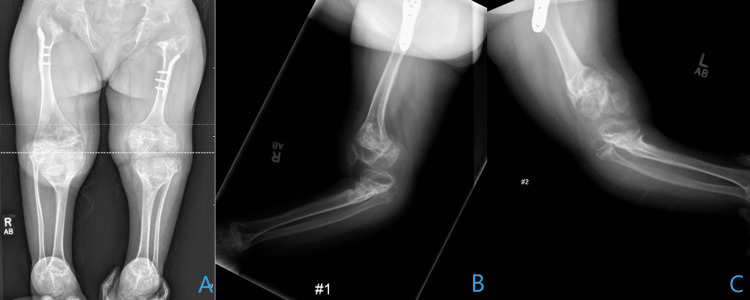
Radiography on initial presentation Anteroposterior (A) and lateral (B, C) radiographs of the lower extremity demonstrate a significant genu recurvatum bilaterally with contributions largely from the tibia on the right (B) and contributions of about two-thirds from the tibia and one-third from the distal femur on the left (C).
